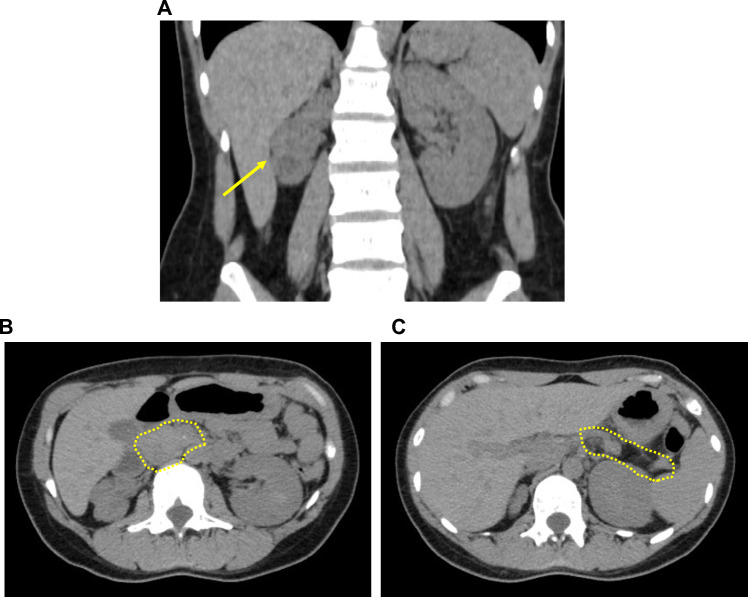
Morphological assessment of the patient’s kidneys and pancreas by CT scanning. A. Both kidneys captured in a coronal section. A yellow arrow indicates the atrophic right kidney. B, C. The agenesis of distal pancreas. The pancreatic head can be captured in a horizontal section (B, indicated by a yellow dotted circle) but the dorsal pancreas cannot be captured in sections with the spleen (the lack of pancreas is indicated by a yellow dotted circle)
